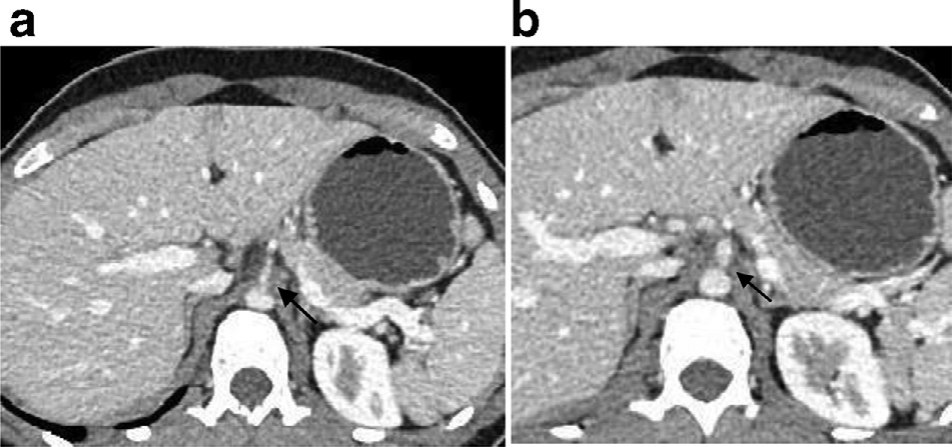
(A): Contrast-enhanced CT axial image of the abdomen shows proximal stenosis of the celiac artery. (B): Contrast-enhanced CT axial image of the abdomen shows proximal stenosis accompanied by post stenotic dilatation, although it is better appreciated on Sagittal CT image.
